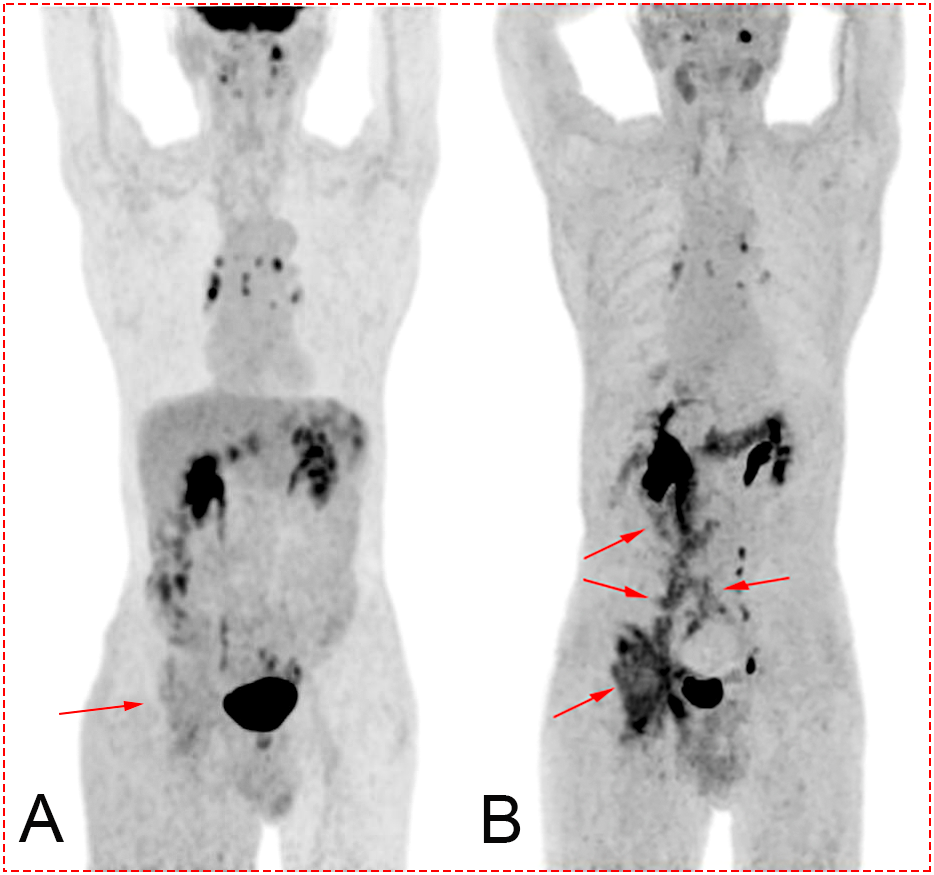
(A) Maximum intensity projection (MIP) after ^18^F-FDG PET/CT. Image of the tumor is not clearly displayed (red arrow). (B) MIP after ^18^F-FAPI PET/CT. The darker sites labeled by the red arrow show the path of metastasis of the tumor from the tumor bed (duodenal stump) to the right groin. Concentration of the tracer mainly located in the biliary tract, pelvis, ureter, bladder, pancreas, and colon.
